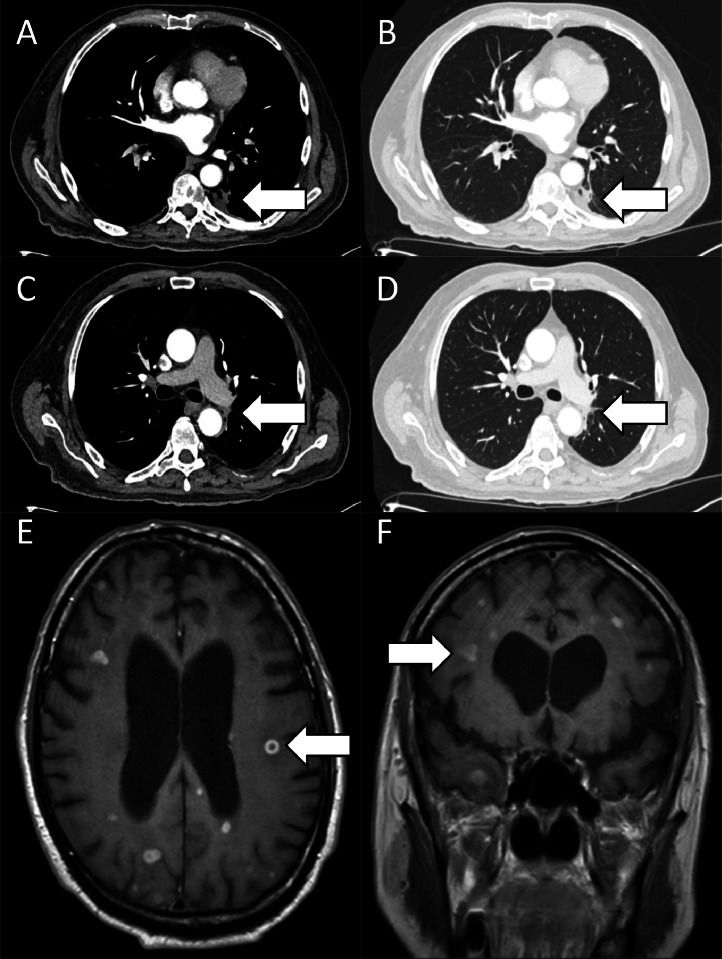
Contrast-enhanced axial CT images of the chest and contrast-enhanced MRI images of the brain taken a month after initiation of treatment.
